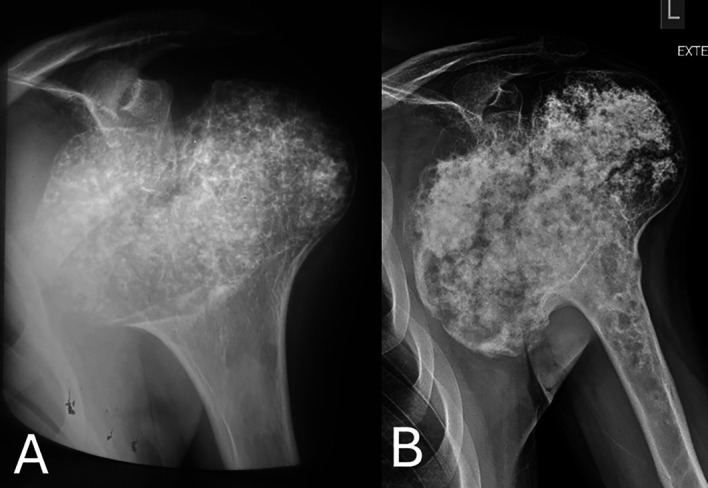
(A) Anteroposterior plain radiograph of left shoulder at the time of initial diagnosis (age 17), showing a bulky bilobed symmetric osteocartilagenous overgrowth of the proximal humeral epiphysis with stippled calcifications, cortical thinning, and a sharp zone of transition towards the metaphysis. (B) Anteroposterior plain radiograph of the left shoulder revealing the bulky, bilobed, incongruent osteocartilagenous overgrowth of the proximal humeral epiphysis. The overgrowth was asymmetric with exuberant enlargement of the medial component, which presented osteolytic areas and areas of cortical breach. Rings and arcs, calcifications, and osteolytic foci were evident in the proximal metaphysis.
